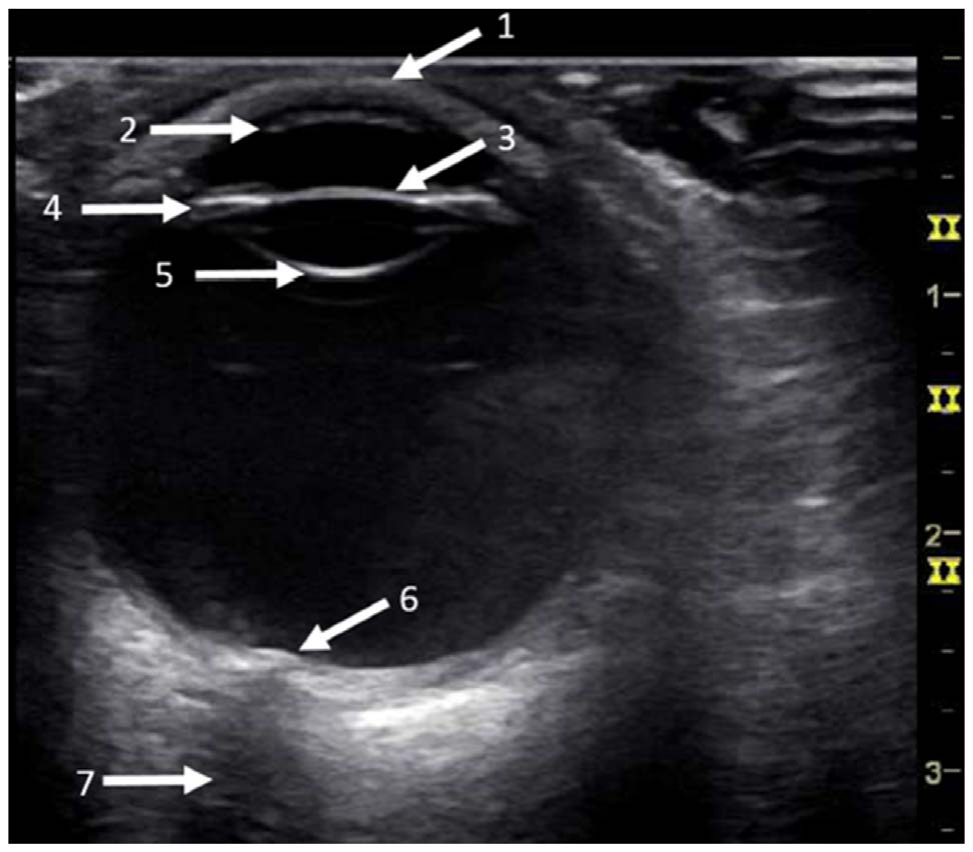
Ocular Ultrasound of a normal eye. The ultrasound probe is applied to the closed eye and transmission gel used to avoid any pressure on the eye. The normal eye (here right eye) appears as a round hypoechoic (grey-black) structure, and the cornea (2) is a thin hyperechoic (white) layer next to the eyelid (1). This is adjacent to the anterior chamber (hypoechoic water-filled cavity). Below the chamber, a hyperechoic iris and ciliary bodies (4) follow with the anterior (hyperechogenic) reflection of the lens (3). The lens itself is hypoechogenic with a smaller reflection on the rear side (5). The vitreous body is hypoechogenic due to its water-filled cavity. Retina may not be differentiated from choroidal layers, while the optic nerve appears as a hypoechoic linear structure (7) entering the vitreous chamber (6).
